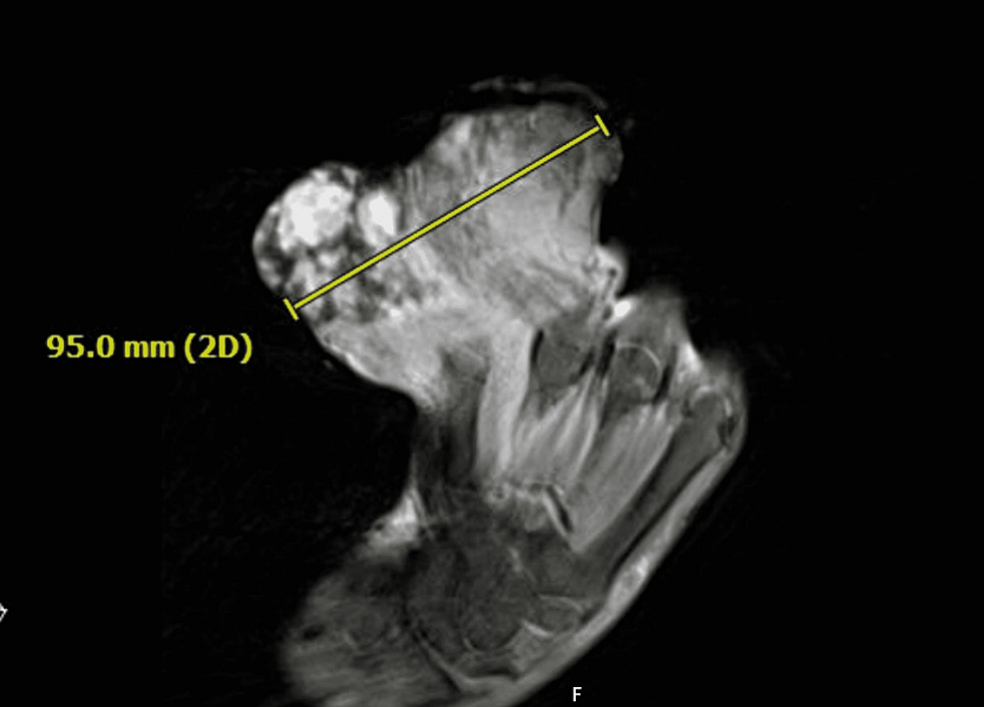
MRI with and without contrast shows a large 9.5cm heterogeneous soft-tissue mass at the dorsal aspect of the fifth digit, with likely involvement of the fifth digit proximal phalanx.
